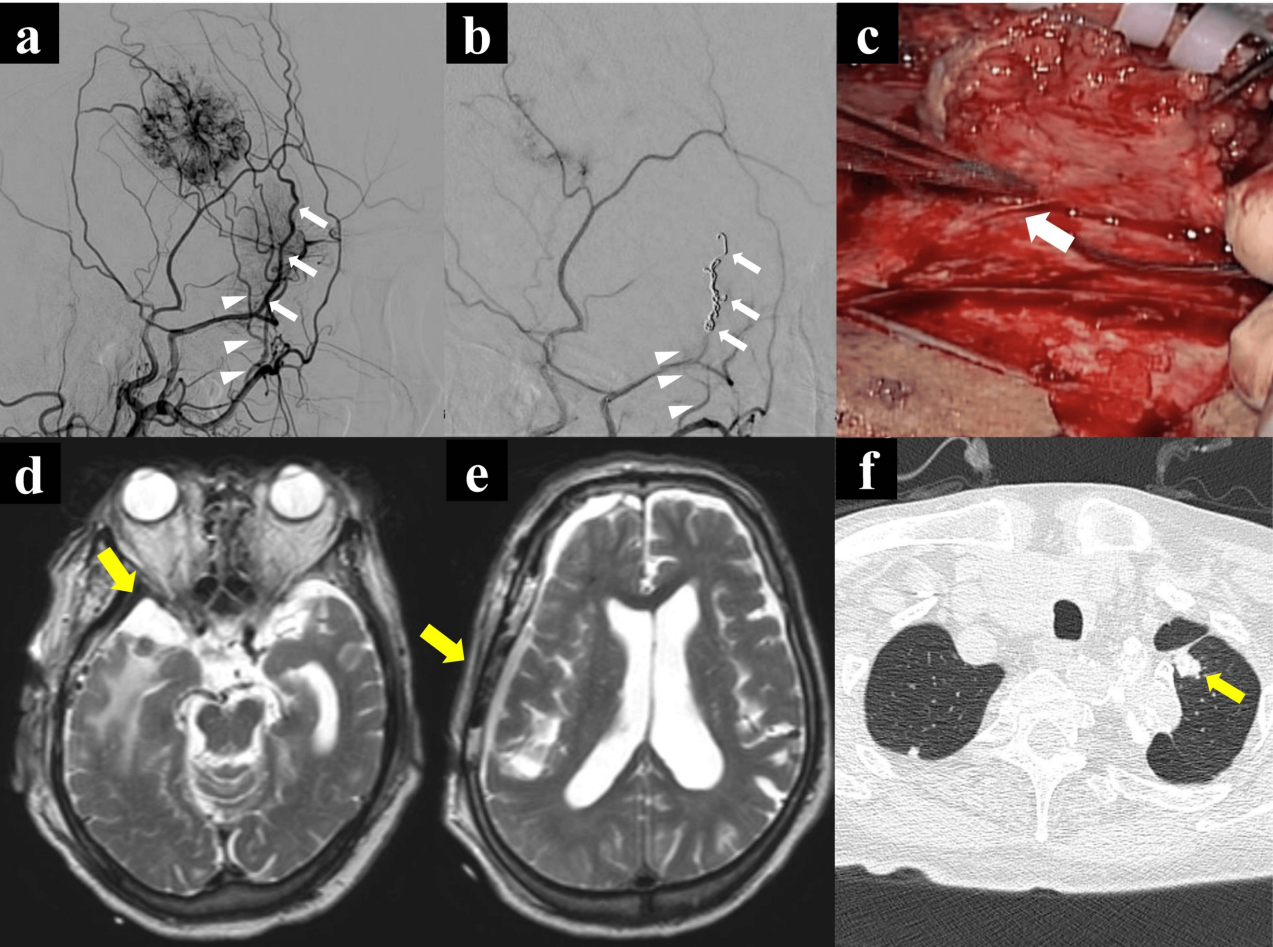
Pre-postoperative digital subtraction angiography (DSA), intraoperative image, and postoperative MRI and CT image DSA of the right internal maxillary artery (IMA) showing that both the right temporal bone tumor and the sphenoid ridge meningioma were supplied with blood from the middle meningeal artery (MMA) anterior branch (a, white arrows). The bone tumor is also supplied by the anterior deep temporal artery (ADTA, white arrowheads). Following preoperative embolization, digital subtraction angiography (DSA) of the right IMA confirmed that the MMA anterior branch was embolized with coils (white arrows), and ADTA was embolized with embosphere microspheres (b, white arrowheads). The temporal bone tumor had blood supply from the MMA during the operation (c, white arrow). Postoperative MRI showed the total removal of sphenoid ridge meningioma (d, yellow allow) and temporal bone tumor (e, yellow arrow). The postoperative systemic radiological examination revealed a metastatic left lung tumor (f, yellow arrow).
